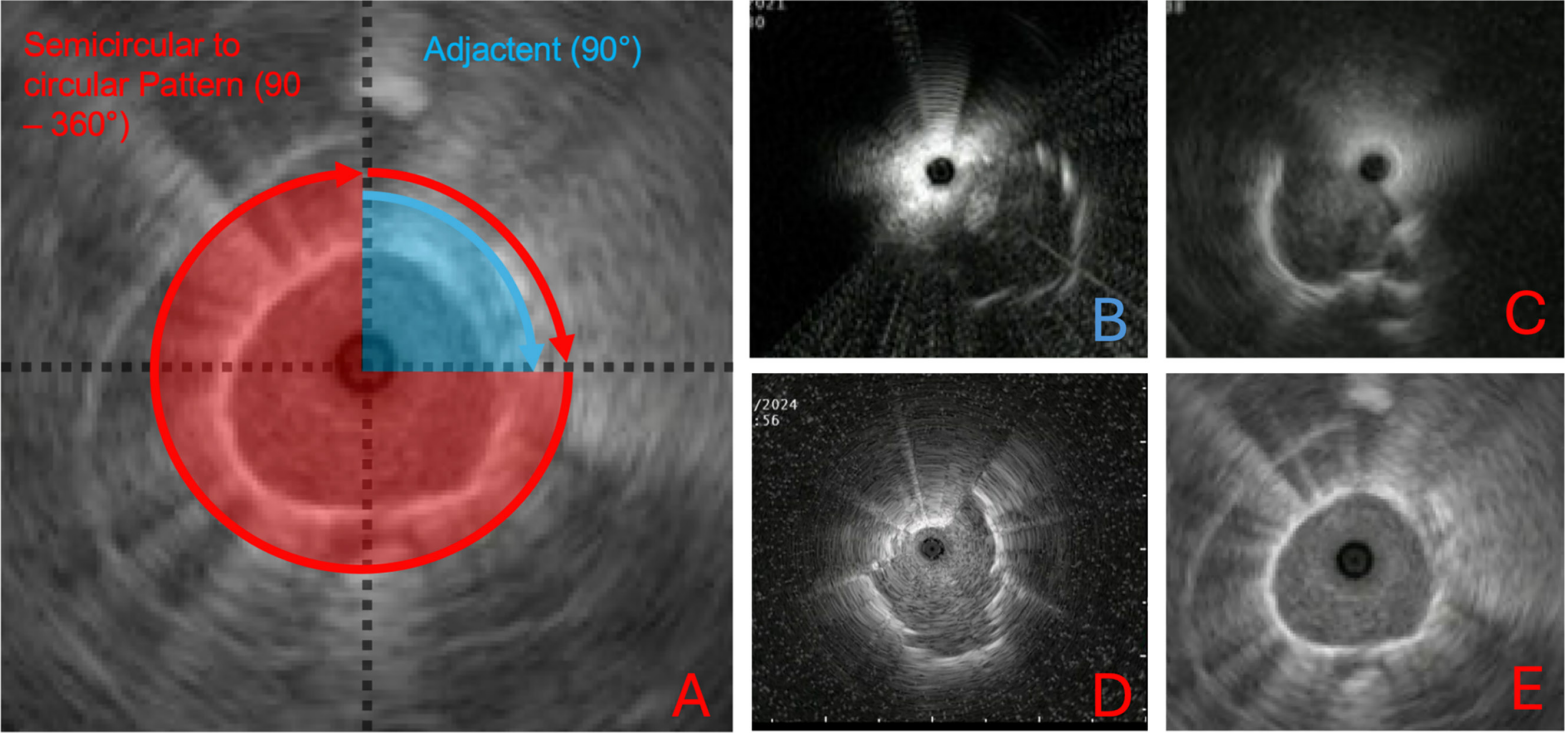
(A) The classification of endosonographic lesion patterns was based on the extent of the lesion signal. Semicircular, three‐quarter circular, and circular signals were collectively referred to as the semicircular to circular pattern (SCP). Eccentric patterns were categorised as follows: Adjacent (up to 90°, B), semicircular (90°–180°, C), and three‐quarter circular (180°–270°, D). A full 360° view was classified as circular (E).
